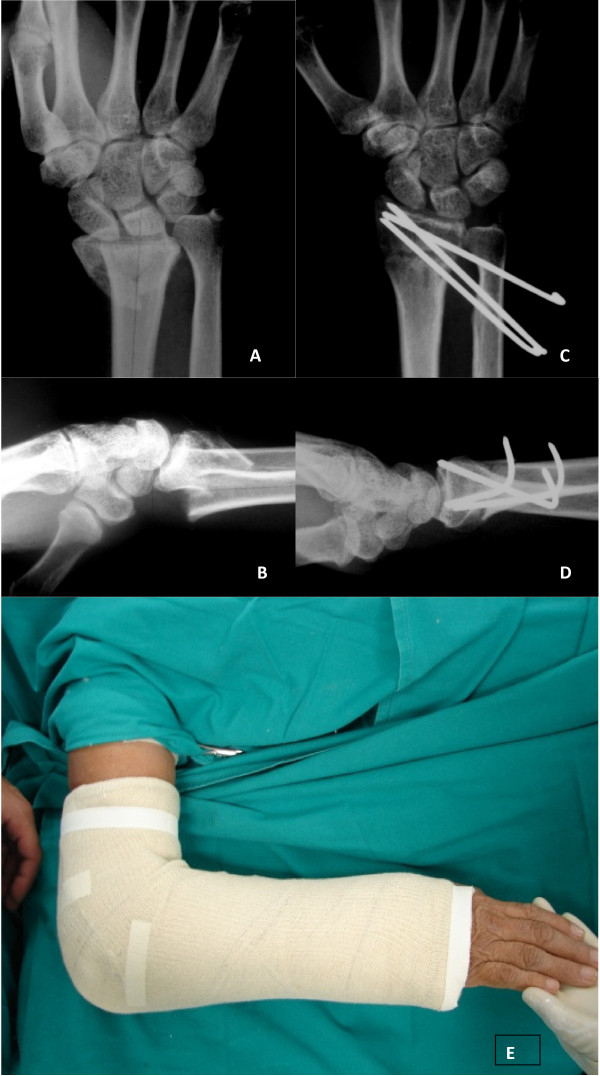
Modified De Palma technique of percutaneous pinning. A. Patient n° 62. Female, 58 years old. Radiograph preoperative in front view. B. Lateral view. C. The fracture of the radius is fixed using the ulna as a support. In the coronal plane we directed the convergent pins towards the styloid process of the radius. D. In the sagittal plane we directed the pin in diverging directions(dorsal and volar). E. Above elbow cast applied post-operative.
